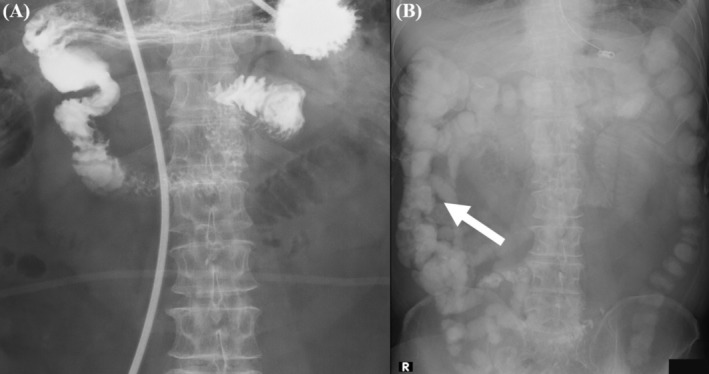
Water‐soluble contrast challenge using Gastrografin. (A) Abdominal radiograph immediately after administration via nasogastric tube. (B) Radiograph obtained 3 h later demonstrates contrast medium reaching the ascending colon (arrow), consistent with type 3b according to the Mori classification, indicating a high likelihood of successful conservative management.
